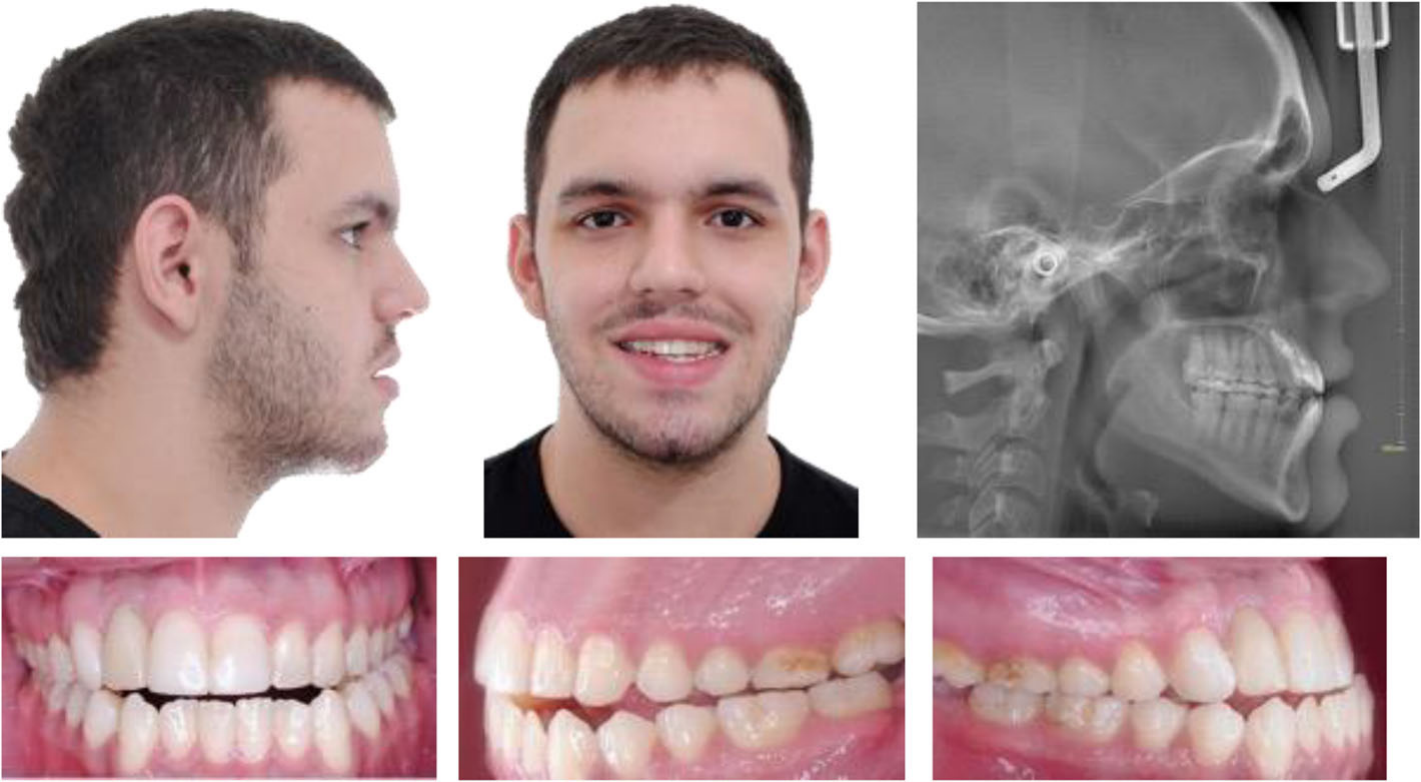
Pre-treatment extra-oral and intra-oral photos, and lateral cephalic X-ray
